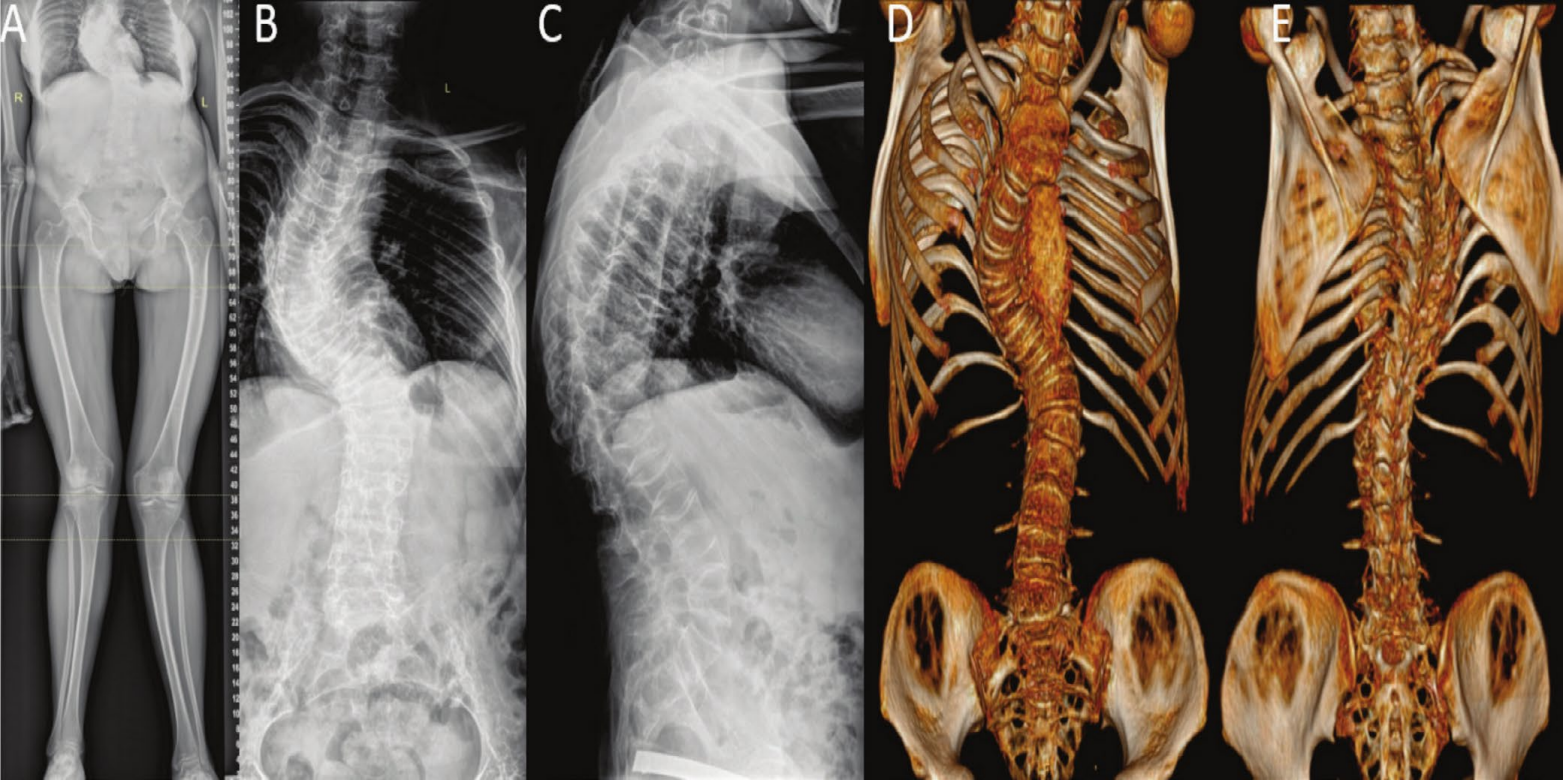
PA (A, B) and lateral (C) radiographs of a 17‐year‐old patient with type IV osteogenesis imperfecta demonstrating a 49° thoracolumbar scoliosis and a 51° thoracic kyphosis with osteopenic, wedge‐shaped, and biconcave vertebral bodies. (D, E) Additional 3D CT images are provided. Images published with permission from patient and parents. IRB approval not applicable.
